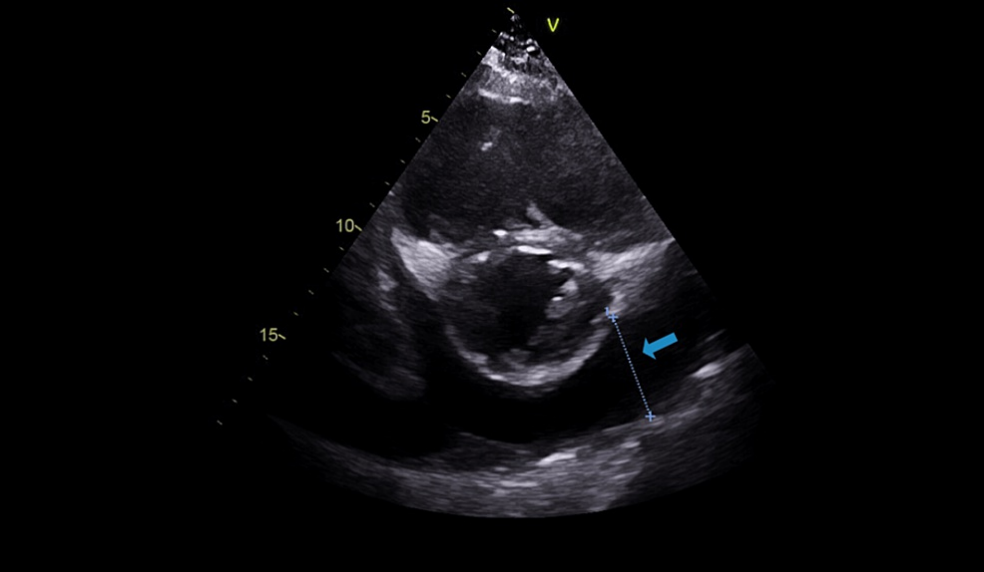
Echocardiogram (image 1) The blue arrow shows pericardial effusion.
